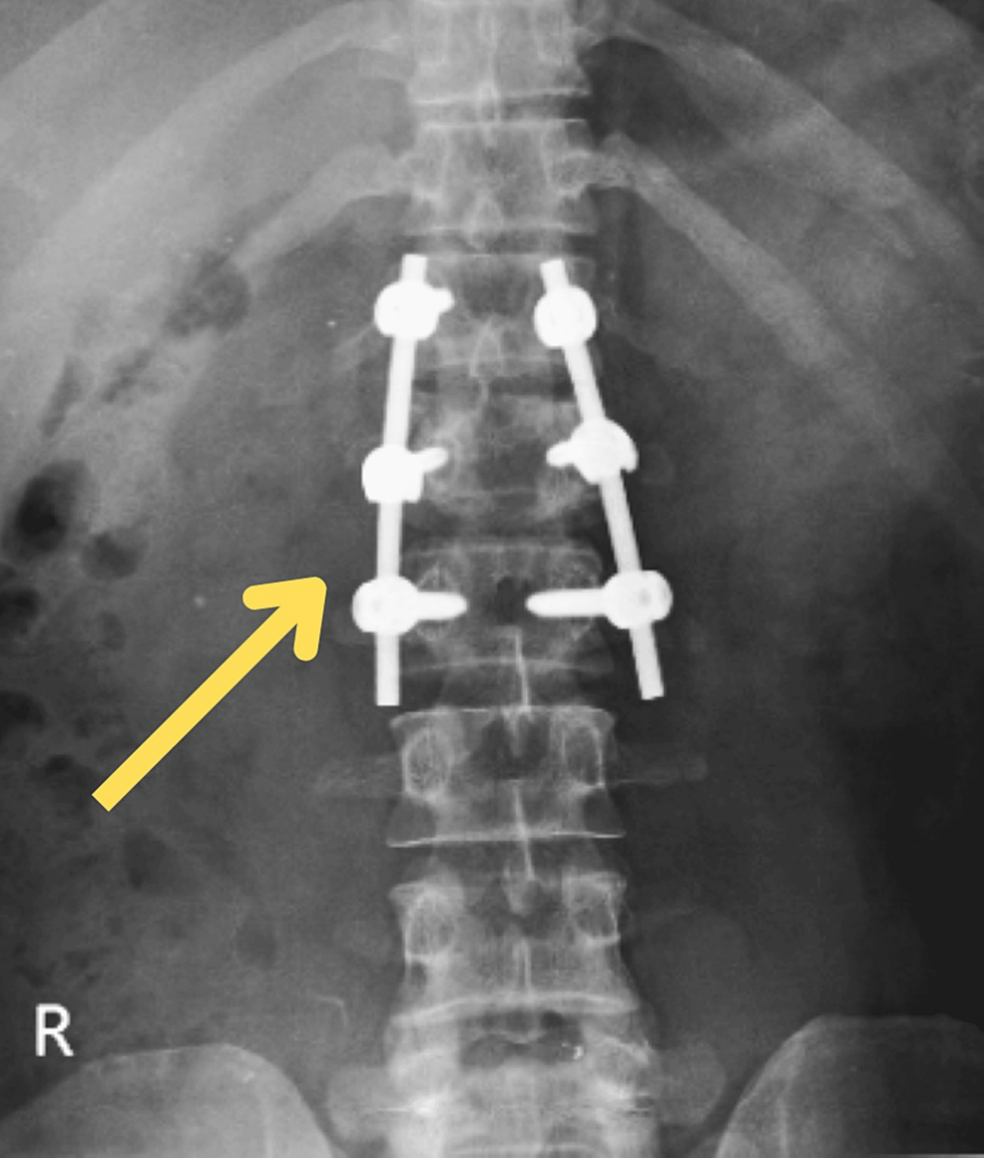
Post-operative X-ray of the spine The yellow arrow points towards the spinal fusion at the D12-L2 level.
